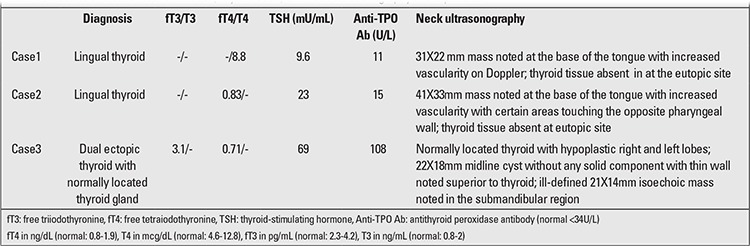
Results of biochemical evaluation, thyroid scan, and neck ultrasonography in the patients
